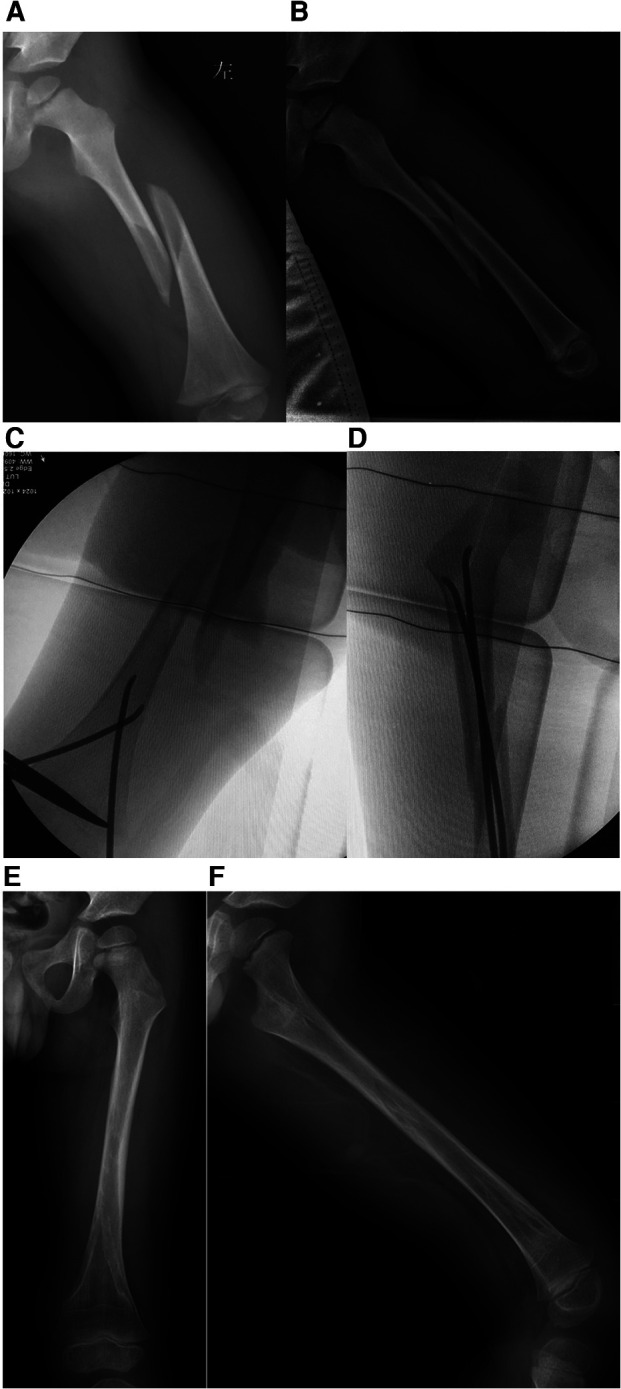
Typical long spiral fracture of left femur of a 3 years old boy. (A) The initial AP x-ray of left femur preoperative. (B) lateral x-ray of left femur preoperative. (C) An entry point was made with the help of a bone awl approximately 2.0–3.0 cm above the physis on the lateral side. (D) C-arm result after closed reduction and ESIN fixation. (E) 9 months follow-up AP x-ray of left femur. (F) 9 months follow-up lateral x-ray of left femur.
